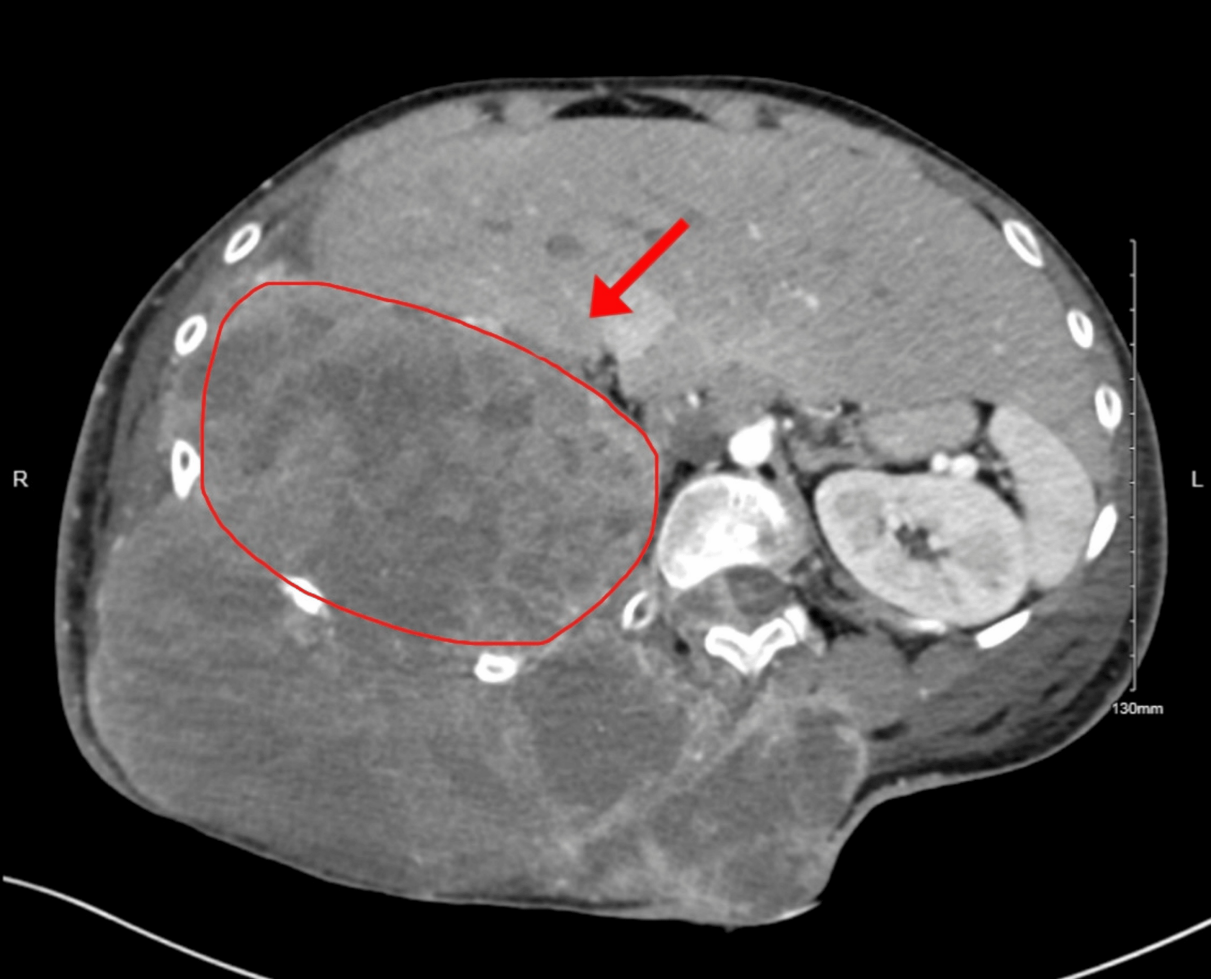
Tthe abdominal CT image demonstrating further inferior extension of the mass into the upper abdomen
